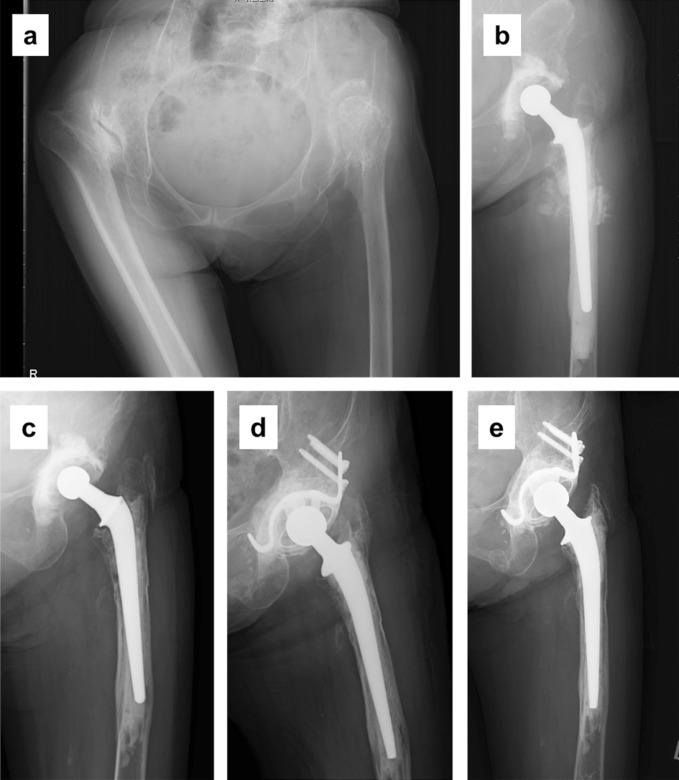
Radiographs of a 69-year-old woman who underwent THA combined with subtrochanteric shortening osteotomy for Crowe IV hip. A, Before surgery and (B) just after cemented THA with osteotomy. C, Apparent stem loosening at 9.5 years after surgery. D, Just after revision surgery with Kyocera type 6 cemented stem. E, No loosening was observed at 9 years after revision surgery. THA = total hip arthroplasty
